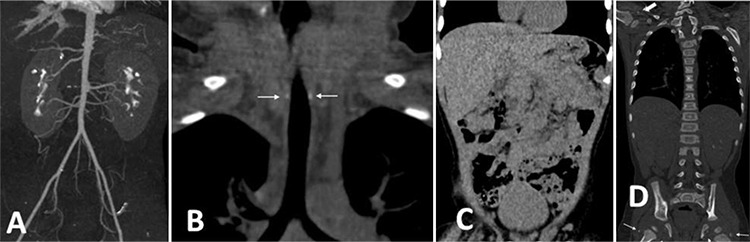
Computed tomography (CT) angiography images and non-contrast CT of abdomen and chest after treatment with magnesium demonstrates: A) Normal appearance of abdominal aorta, bilateral internal and external iliac arteries, femoral artery, renal and mesenteric arteries, B) At the level of the larynx, soft tissue calcifications are reduced in the paratracheal region and around the hyoid bone. C) Mesenteric artery wall calcifications are not observed. D) Periarticular calcification in the right shoulder joint and in the right hip joint are reduced
